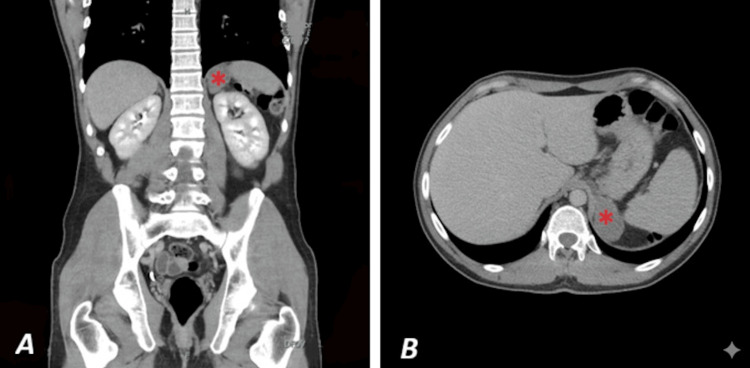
Thoracic contrast-enhanced computed tomography (CT) scan A multilobulated mass-like process with a few small calcifications was observed in the left adrenal compartment, measuring 48 × 31 mm in the axial plane, appearing to extend toward the esophagogastric junction. This lesion demonstrated mild enhancement after intravenous contrast administration, increasing from 24 HU to 42 HU on the portal venous phase. The fat plane was focally disrupted between the mass and the stomach on the one hand, and between the mass and the left adrenal gland on the other. However, this mass did not primarily appear to be of adrenal origin. A. Bronchogenic cyst (*) on CT-scan in coronal plane B. Bronchogenic cyst (*) on CT-scan in axial plane
